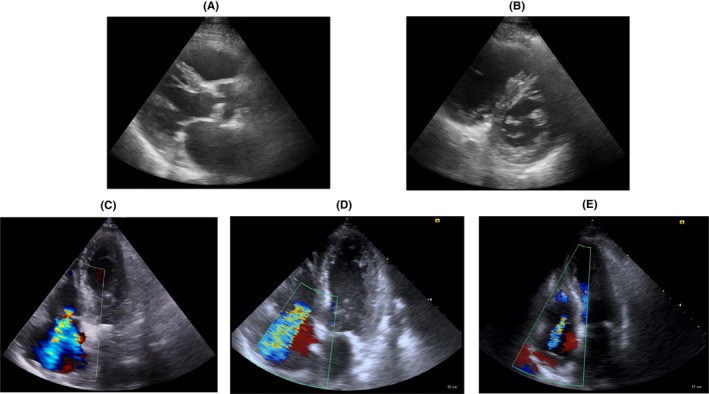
Transthoracic echocardiography. (A) Parasternal long‐axis view at the presentation (Day 1) (B) Apical 4‐chamber view at the presentation (Day 1) (A) and (B) show a significantly dilated right ventricle, suggesting right ventricular volume overload. (C) Tricuspid regurgitation (TR) jet at the presentation (Day 1). (D) TR jet 5 h after the presentation (Day 1) shows exacerbation. (E) TR jet on Day 5 shows marked improvement.
